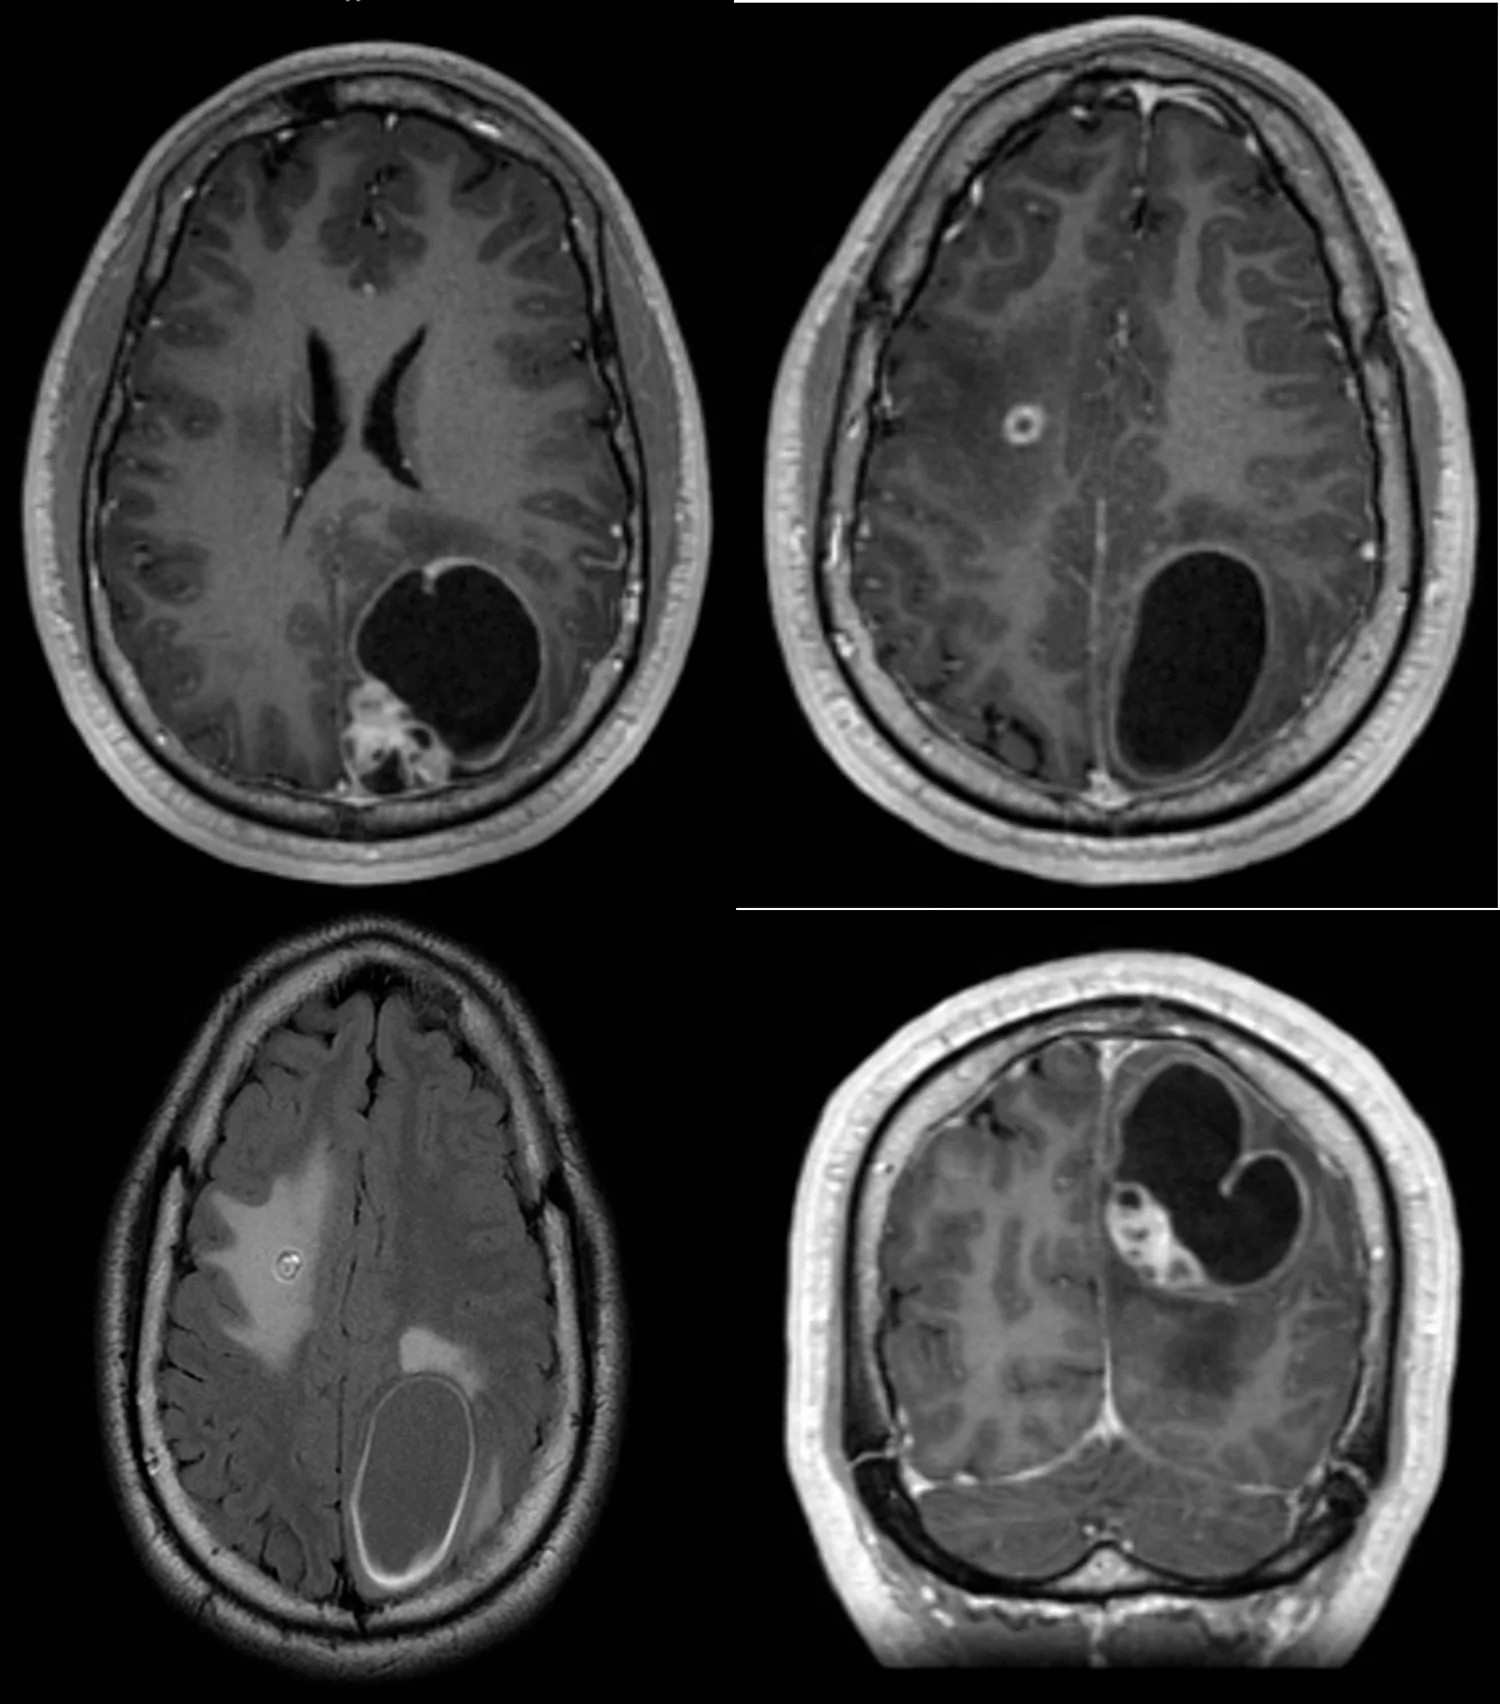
Patient’s initial MRI of the brain (bottom left: T2 FLAIR sequence, all other images T1 post-contrast sequences) further characterizing the left occipito-parietal lesion as mixed cystic and nodular with avid contrast enhancement and mild surrounding vasogenic edema with the ring-enhancing right centrum semiovale mass
